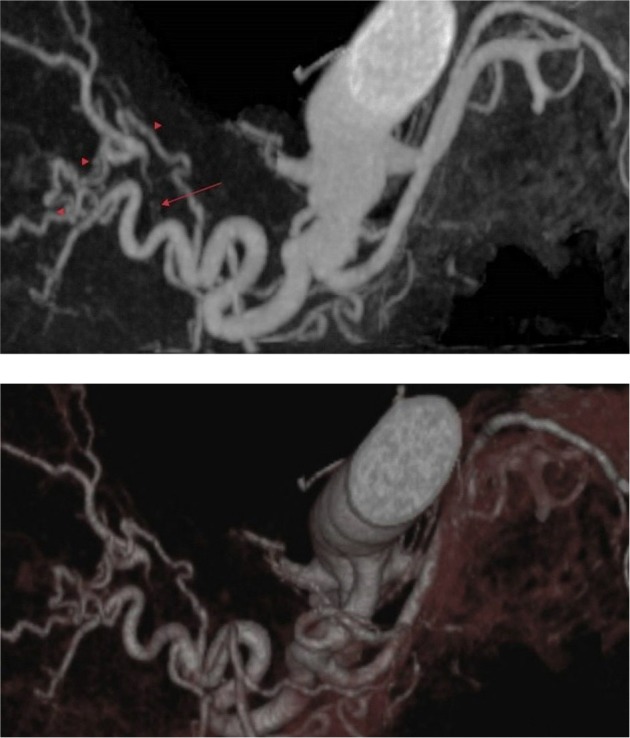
Thrombosis in the left hepatic artery. Thrombosed segments and collaterals can be seen on the MIP and VRT images of the patient who are thought to be patented by Doppler USG due to collaterals. (a) A reconstructed MIP image demonstrates thrombosis (arrow) at the proximal segment of the left hepatic artery and distal reperfusion with collateral branches (arrowheads), (b) VRT image of the same patient.
